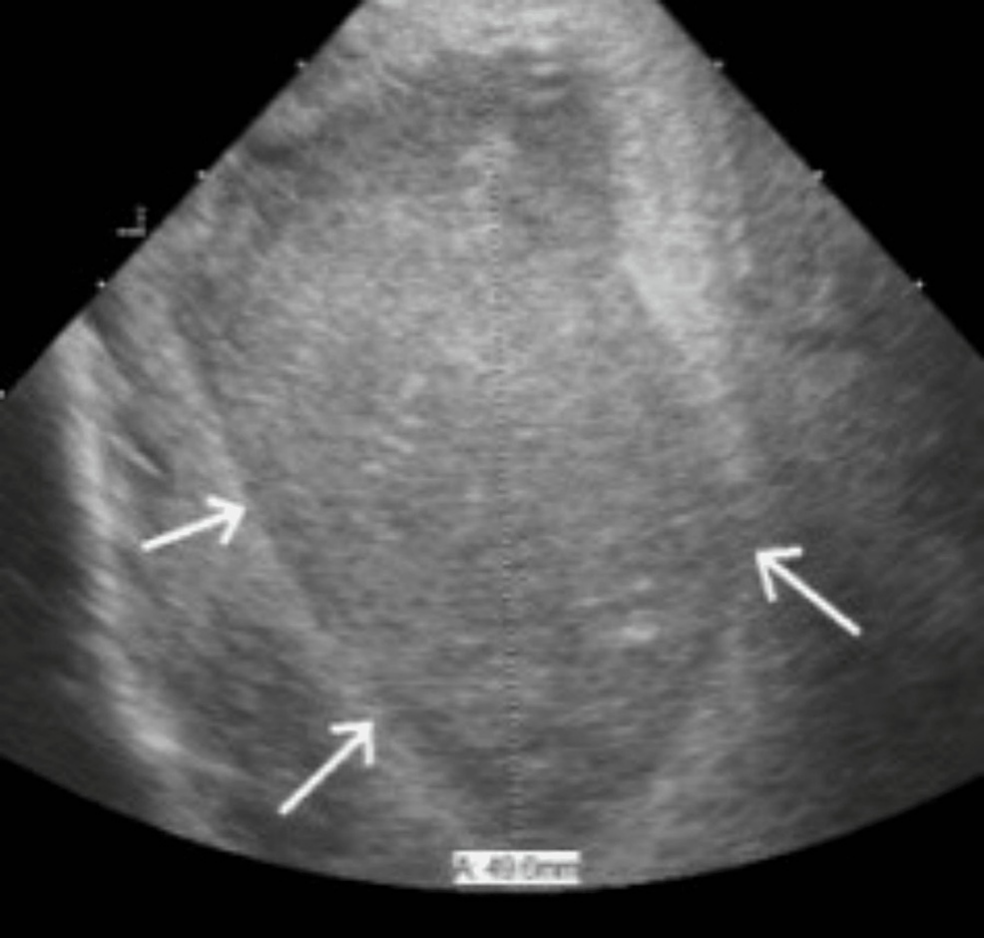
Intraoperative-focused ultrasound of right frontal lobe demonstrating frontal lobe abscess (arrows) from frontal sinus disease, measuring 5 cm in diameter.
